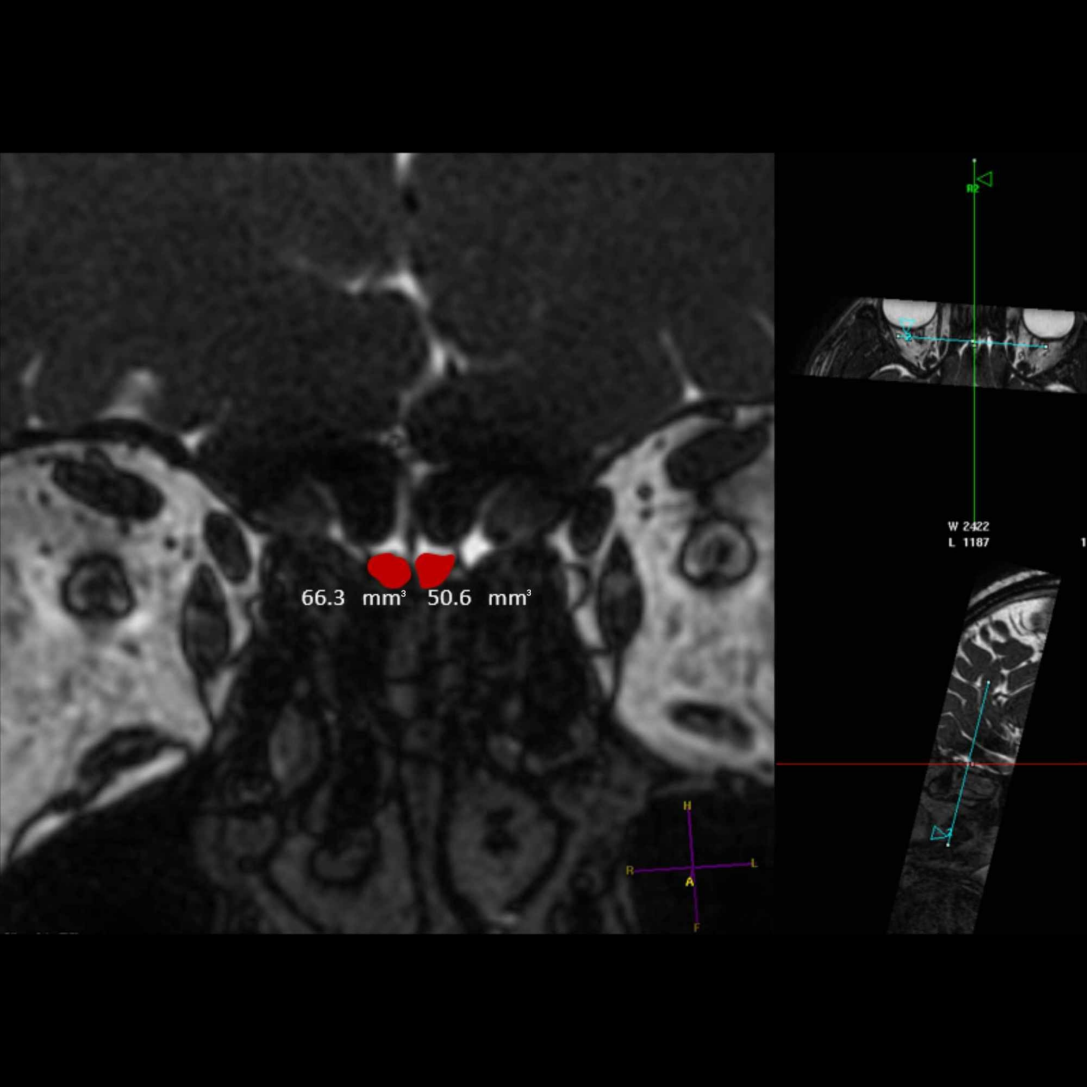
Coronal T2-weighted image Semi-automatic measurement of OBV on the MPR 3D workstation using manual segmentation. To minimize the possibility of errors in volumetric measurements, the images were magnified as much as possible. Both OBs are shown in red and their measured volumes are given underneath in mm^3^. OB: olfactory bulb, OBV: olfactory bulb volume; MPR: multiplanar reformation; 3D: three-dimensional
